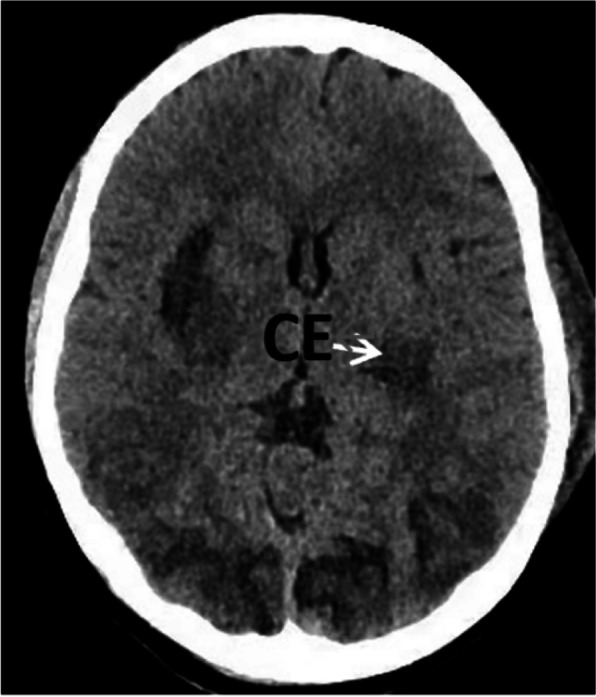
Head CT revealing extensive narrowing of the cerebral sulcus and a decrease in the density of the bilateral basal ganglia and centrum semiovale, indicating cerebral edema
